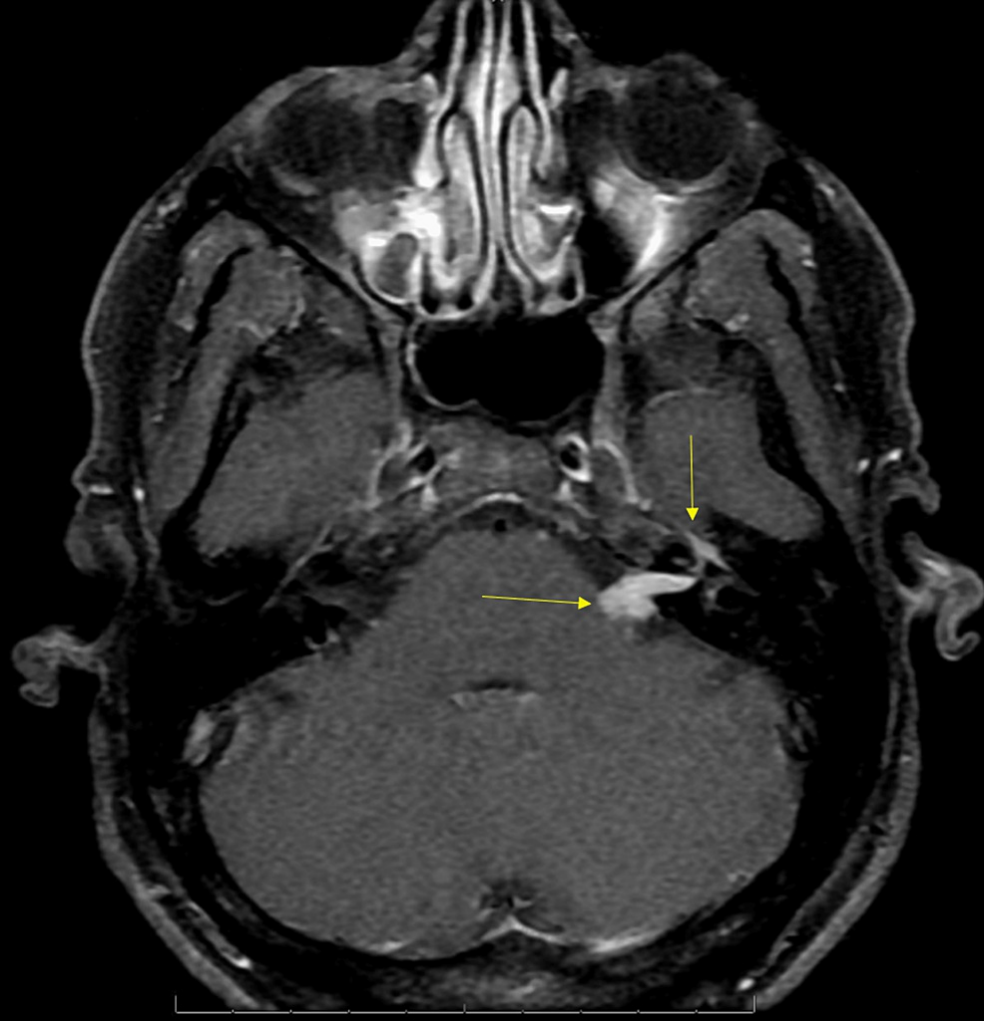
Initial axial T1 fat-saturated sequence MRI of the brain The image shows a mass-like enhancement of the left internal auditory canal, geniculate ganglion, and left seventh cranial nerve (seen by arrows above) consistent with the initial diagnosis of schwannoma.
